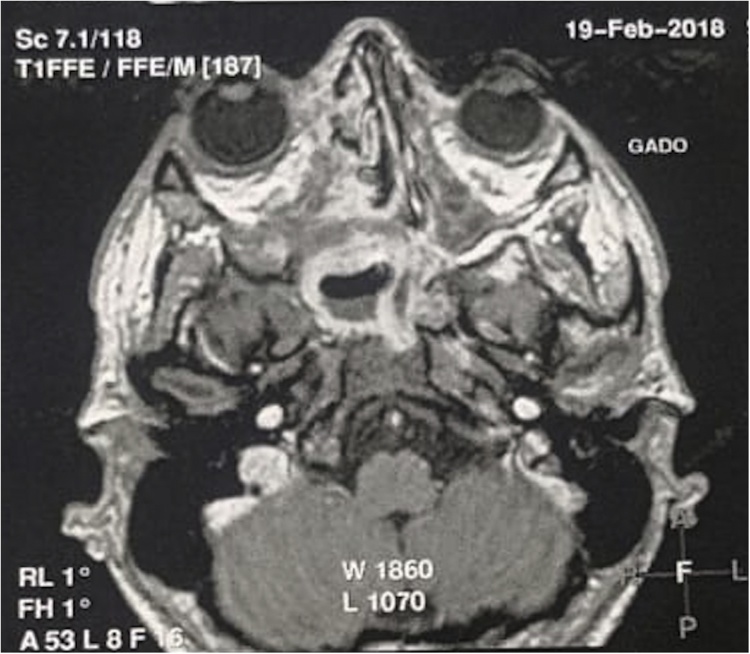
Axial image of MRI showing regression of 50% of the tumor after radiation therapy and chemotherapy.
